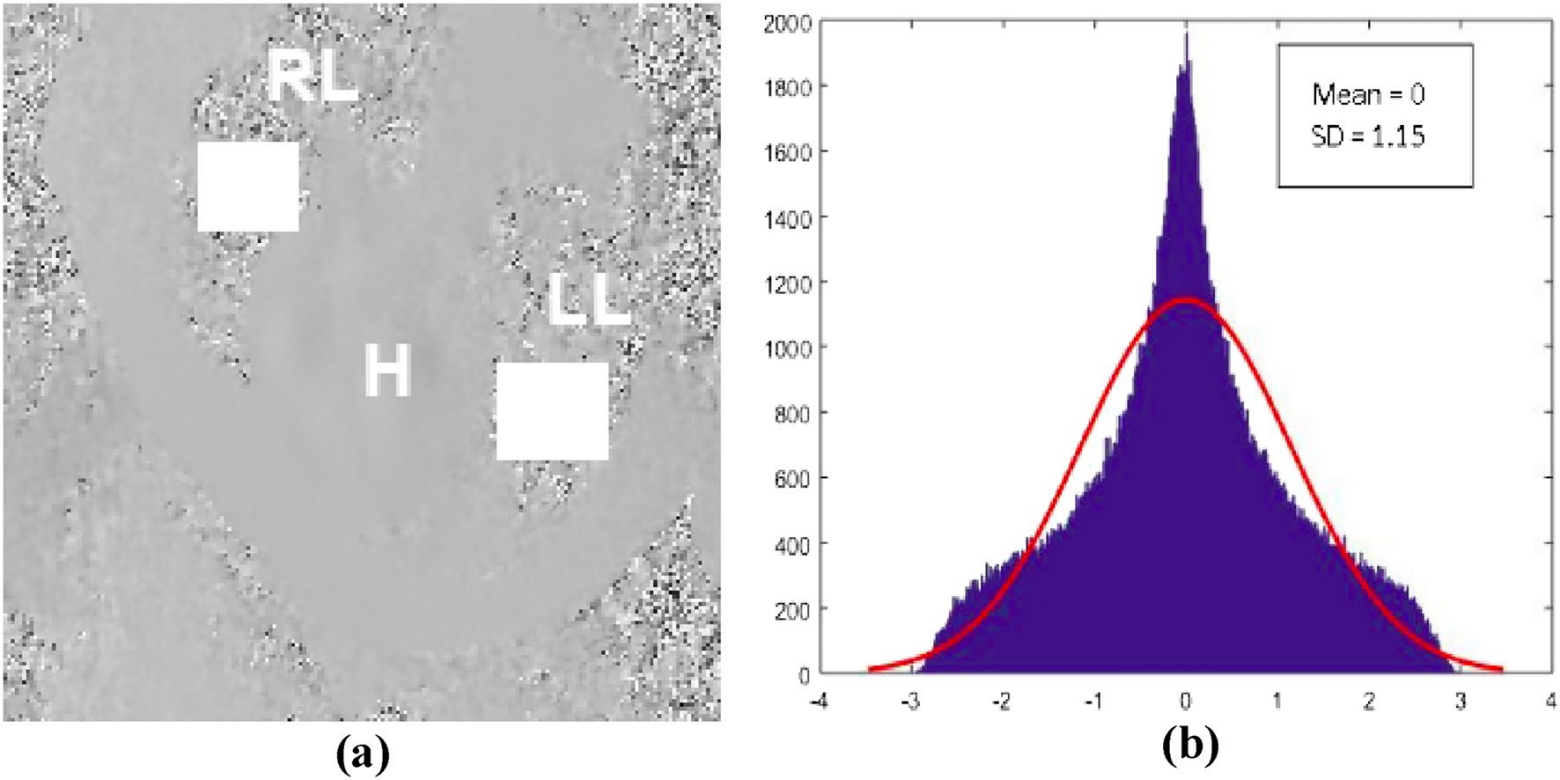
Determination of nature of noise present in PC MRI Data (a) Segmentation of a rectangular region from two lungs. (b) Fitting of a normal distribution to the histogram of the segmented pixel values. RL represents the location of the right lung, LL represents the location of the left lung, H represents the location of the heart.
